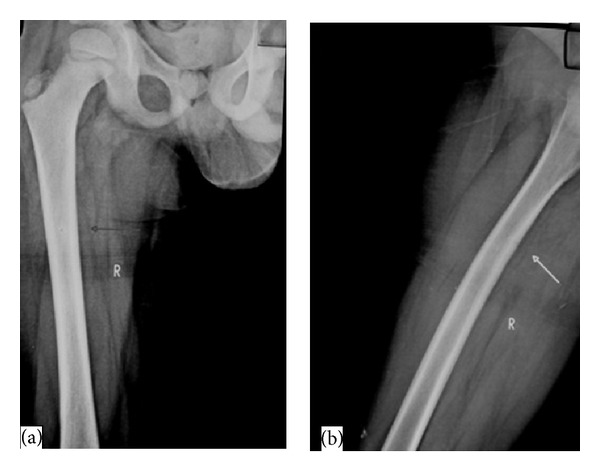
Plain radiograph AP (a) and lateral (b) views of the right femur done after 6 weeks show no significant change in the appearance of lucent bony defect noted along the medial cortex of the right femur.
